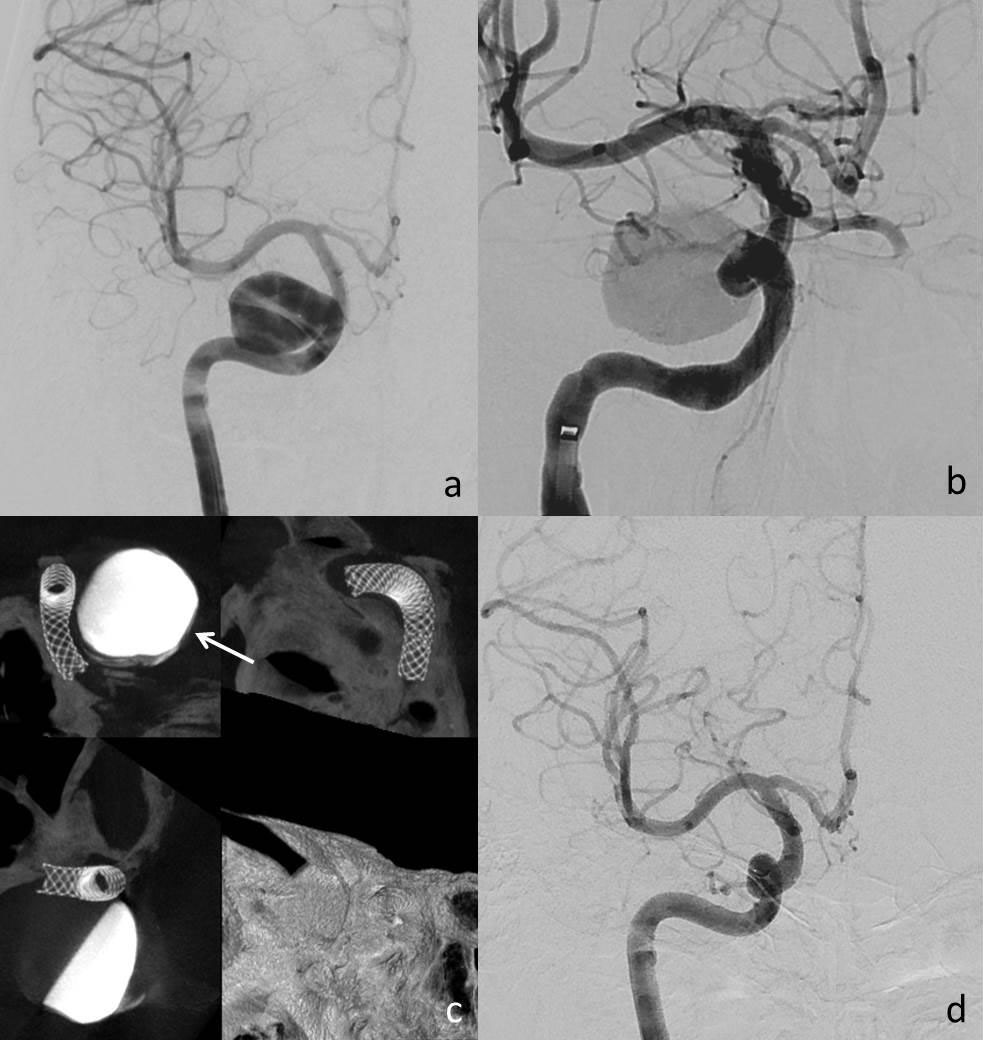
A 21-year-old man previously treated using a detachable balloon (GOLD BAL1, Balt Extrusion, Montmorency, France). a) Follow-up revealed a dissecting aneurysm 14 × 15 mm in size in the right ICA cavernous segment. b) A 4.5 × 16 mm PED Flex was deployed into the ICA, and flow into the aneurysm was markedly slower after the procedure. c) Dyna CT images show a well-opened stent and contrast medium stagnation (arrow) in the aneurysmal sac. d) A first-year control DSA image demonstrating complete occlusion of the aneurysm and full patency of the stent.
